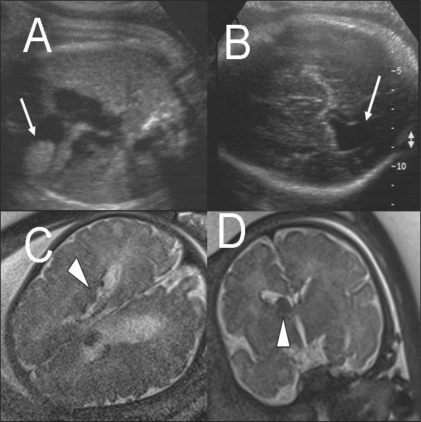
Single live gestation at 37 weeks. USG scans of the heart (A) and brain (B) show a cardiac rhabdomyoma (arrow in A) and mild ventriculomegaly (arrow in B). Axial (C) and coronal (D) T2W MRI images show subependymal tubers (arrowheads), confirming the diagnosis of tuberous sclerosis. The large left lateral ventricular subependymal nodule at the foramen of Monroe is probably responsible for the unilateral ventriculomegaly. The infant developed ash-leaf macules at 1 month and myoclonic jerks at 2 months of age. It died at 3 months (cradle death)
